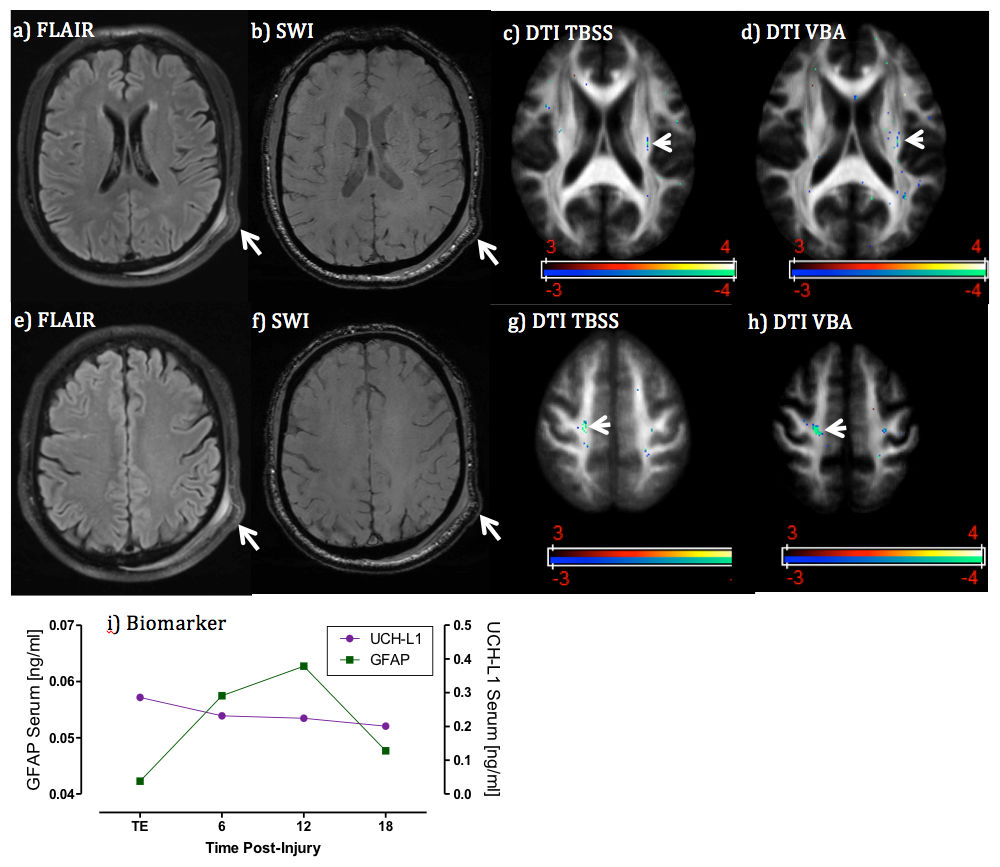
Case 2. MRI and biomarker profile in a patient with traumatic axonal injury but normal-appearing structural MRI. Panels a-d) are MRI images at the corpus callosum and fornix level. Panels e-h) are MRI images at the level of superior coronal radiata. Panel i) is blood biomarker profile. FLAIR and SWI images both indicate the skalp contusion at the parieto-occipital region (long arrows) but normal-appearing brain structure. However, both DTI TBSS and VBA analyses detected significantly reduced FA values at the ipsilateral side (corticospinal tract) and contralateral side (superior corona radiata) of brain white matter (arrow heads), in suggestion of coup and contra coup injury at the microstructure of white matter. Cold color indicates reduced FA values in comparison with controls (t>3 for t-test). Blood biomarkers indicate slightly increased GFAP levels over time but significantly increased UCH-L1 at the admission.
